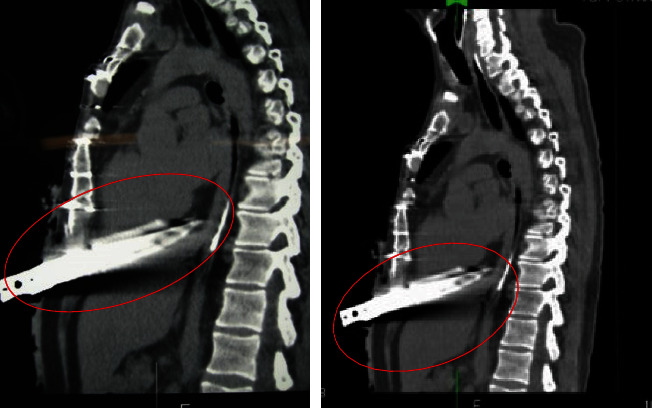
Lateral view of the chest on CT examination. The knife is represented by the red circle.
